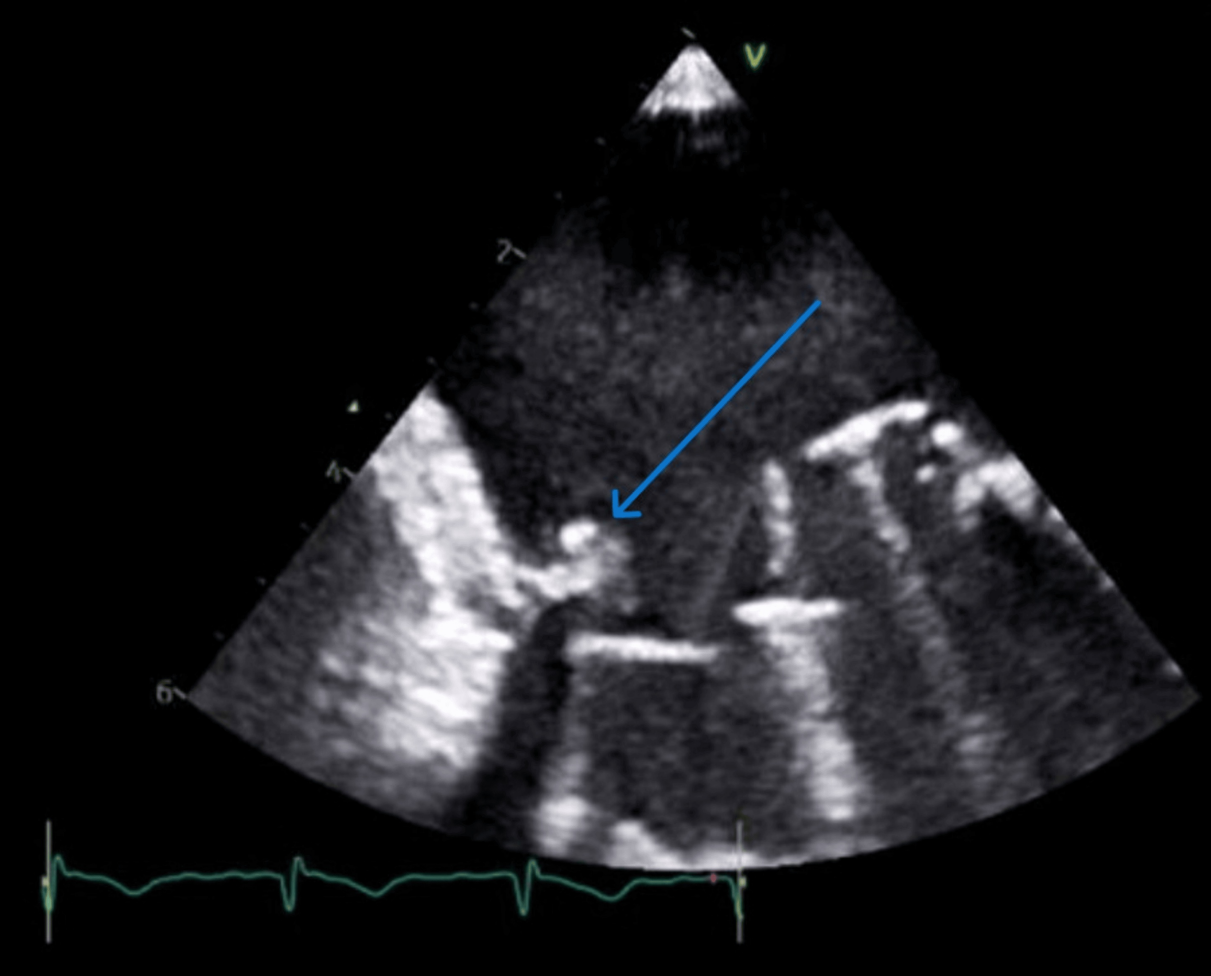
TEE showing 7x3mm vegetation attached to the metallic mitral leaflet TEE: transesophageal echocardiogram
